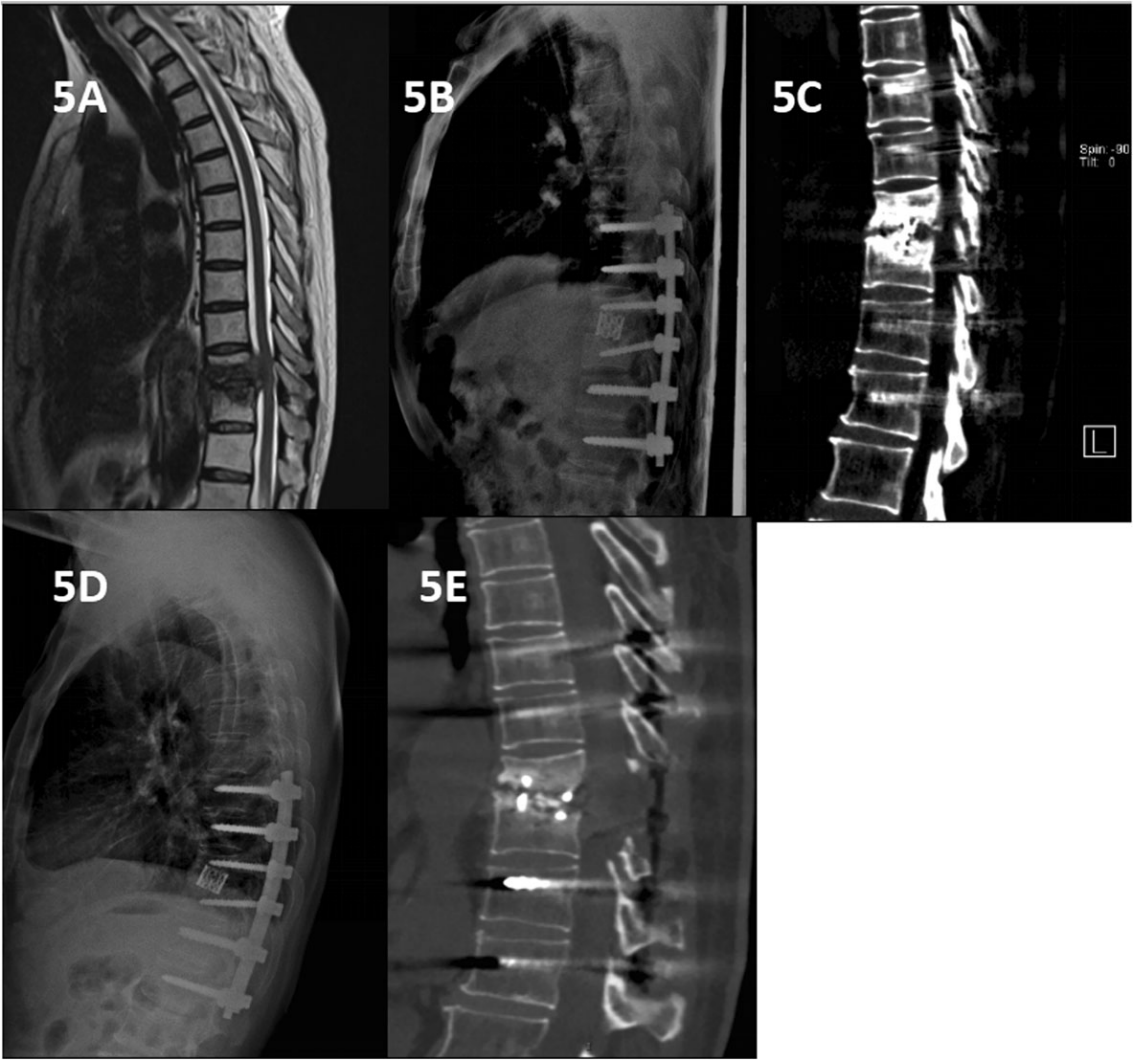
A 42-year-old man with thoracic spinal tuberculosis (T11–12) underwent posterior debridement and decompression combined with internal fixation. a Pre-treatment MRI showing the destruction of the T11–12 vertebrae and concomitant compression of the spinal cord. b Immediate postoperative radiographs demonstrating posterior debridement, bone graft and internal fixation. c, d, e At the 24-month follow-up, plain X-ray and CT showed maintenance of the correction and solid fusion, good clinical effect and subsidence of the titanium mesh cage
